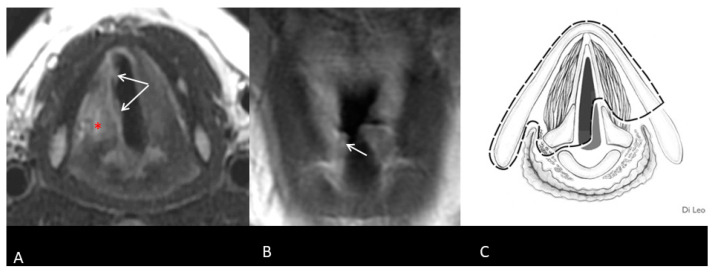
Recurrent right glottic SCC after TOLM: MR sequences FSE T2-weighted on the axial plane (A) and FSE T1-weighted after contrast injection on the coronal plane (B). An irregular thickening (arrows) of the right side of the glottic level is suspicious for tumor. Oedematous changes are present (asterisk) (A). The lesion does not involve the subglottic region (B). Axial scheme of resection (Type IIa OPHL + right ARY) (C) [10].
